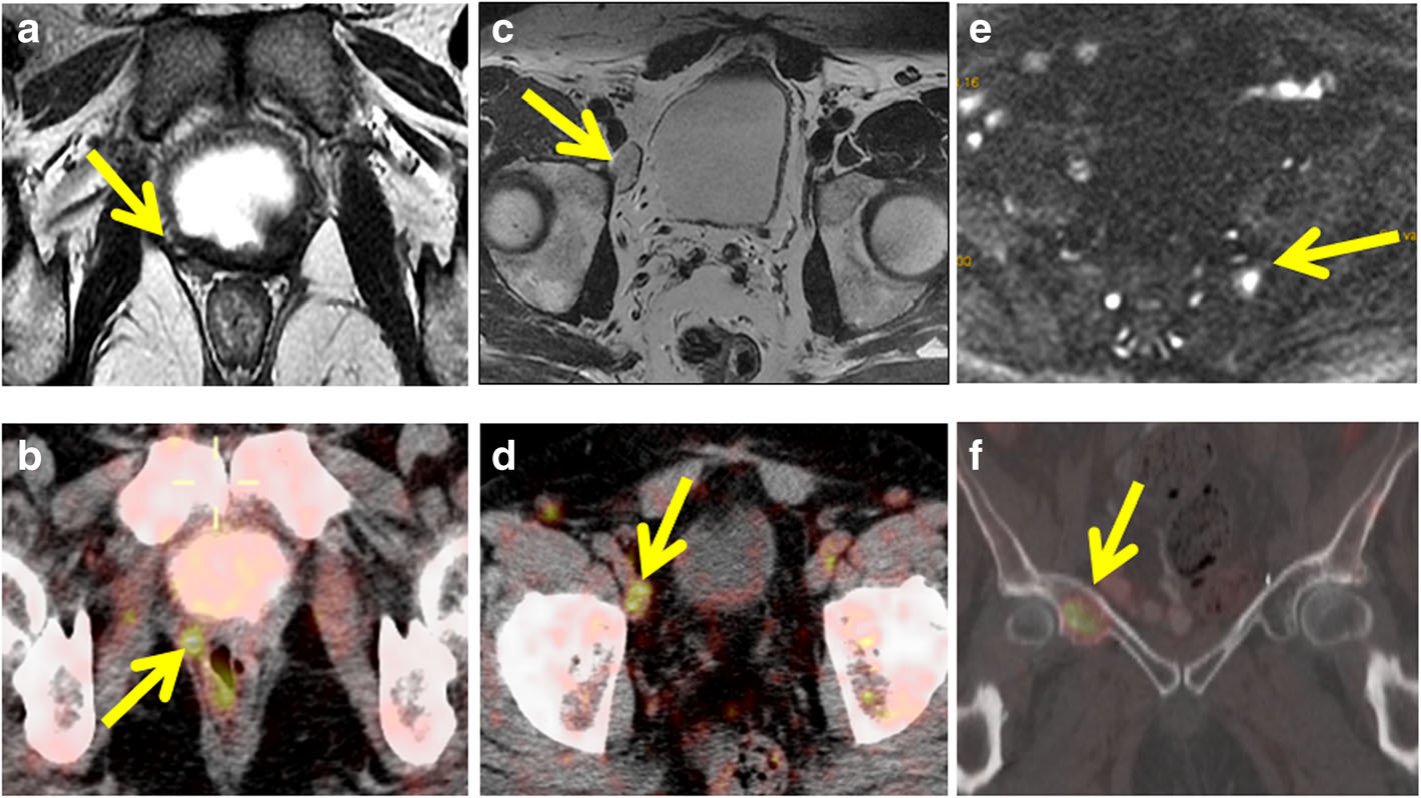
Representative images of different pelvic tumor recurrences detected by mpMRI and 18F-CH PET/CT (*arrows*). a Local recurrence in axial T2-weighted MRI and b local recurrence in 18F-CH PET/CT. c Right external iliac lymph node detected by axial T2-weighted MRI. d 18F-CH PET/CT image at corresponding level demonstrates choline-avid right external iliac lymph node. e Axial Diffusion Weighted Imaging (DWI) of bone metastases in left sacrum (*arrow*) and; f 18F-CH PET/CT shows a hypermetabolic bone metastases in right acetabulum
